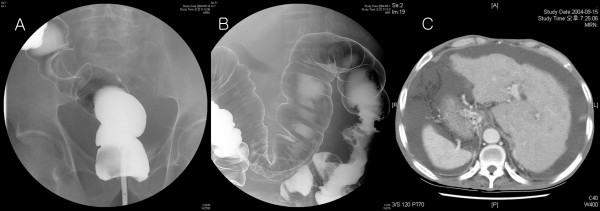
Synchronous double primary cancer in a 66-year-old male patient with situs inversus totalis.a Barium double contrast study showing an ulcerative tumor in the sigmoid colon. b Barium double contrast study showing an ulcerative tumor in the proximal transverse colon. c An abdominal computed tomography scan shows liver cirrhosis with splenomegaly and a large amount of ascites.
